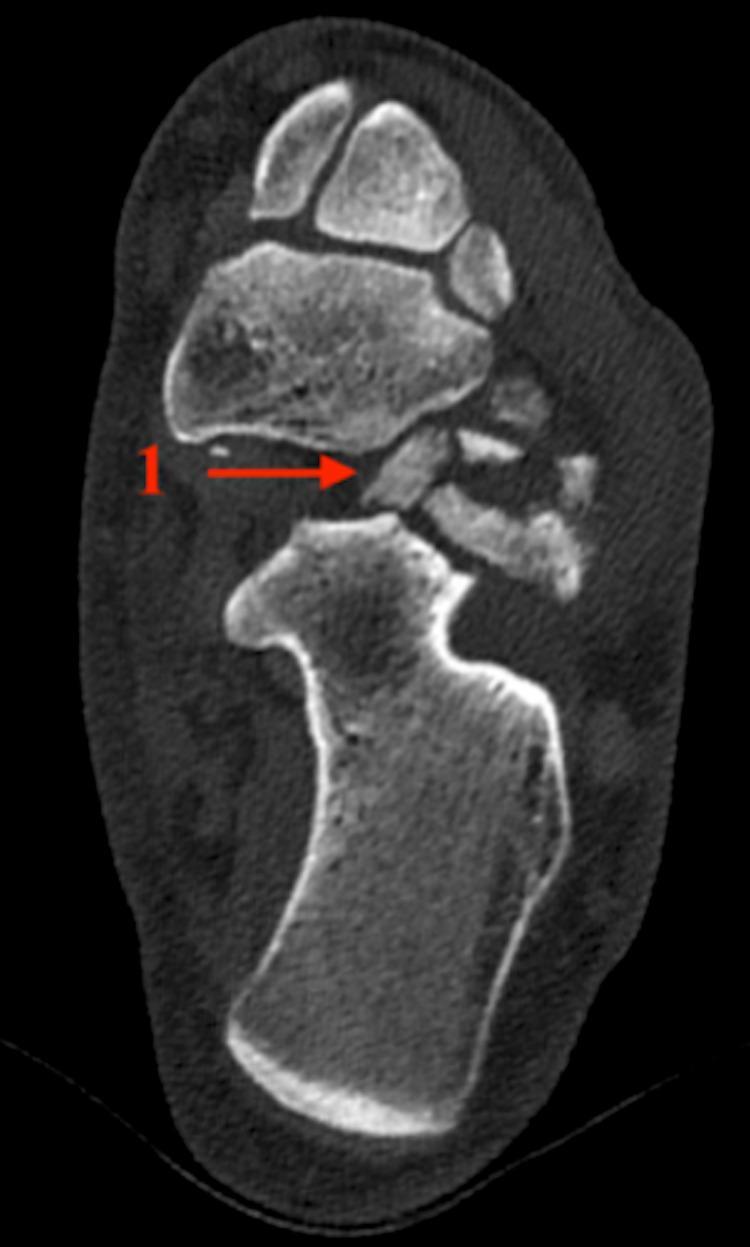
CT axial view demonstrating cuboid comminution 1: Cuboid comminution.
